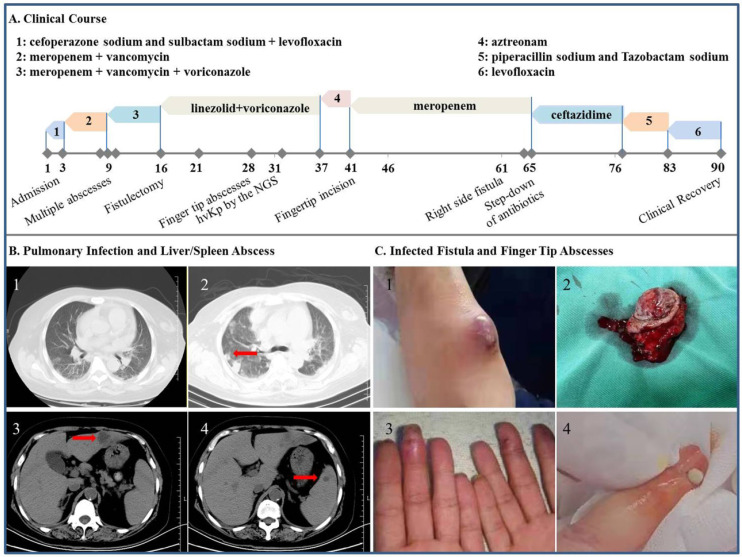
Clinical, imaging and morphologic characterization in the patient with hvKp infection. A: Clinical course of the patient's hospital stay. B1: Chest CT scan at admission. B2-4: CT scan on hospital day 9 showing abscess of the lung, liver and spleen (arrow), respectively. C1 and C2: Tumoral dilation and purulent exudation of the arteriovenous fistula, prior to and after the fistulectomy, respectively; C3 and C4: scene reconstruction of the fingertip abscesses.
